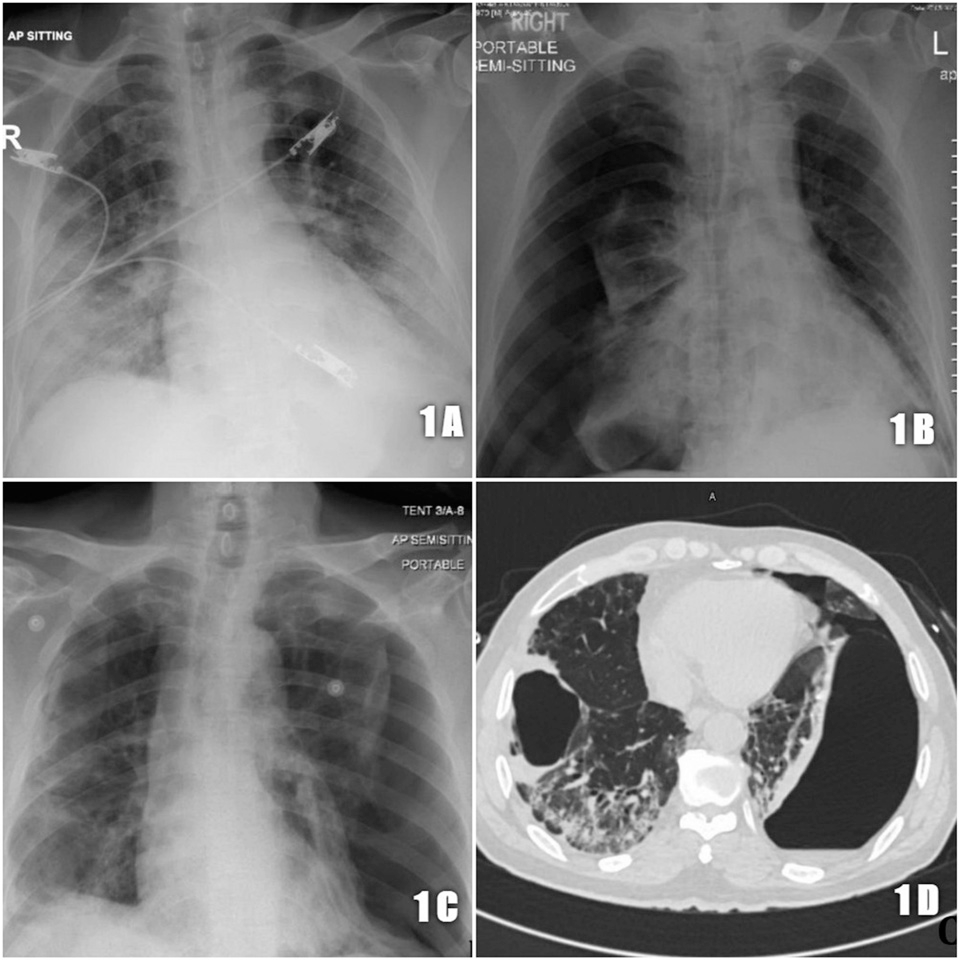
(A) Chest X-ray depicting bilateral lung infiltrates, (B) chest X-ray depicting right pneumothorax with right lung collapse and tracheal deviation to the left, (C) chest X-ray depicting bilateral pneumothorax with left > right and tracheal deviation to the right, and (D) chest CT depicting bilateral bullae, ground-glass appearance, and large left pneumothorax with underlying collapsed lung.
